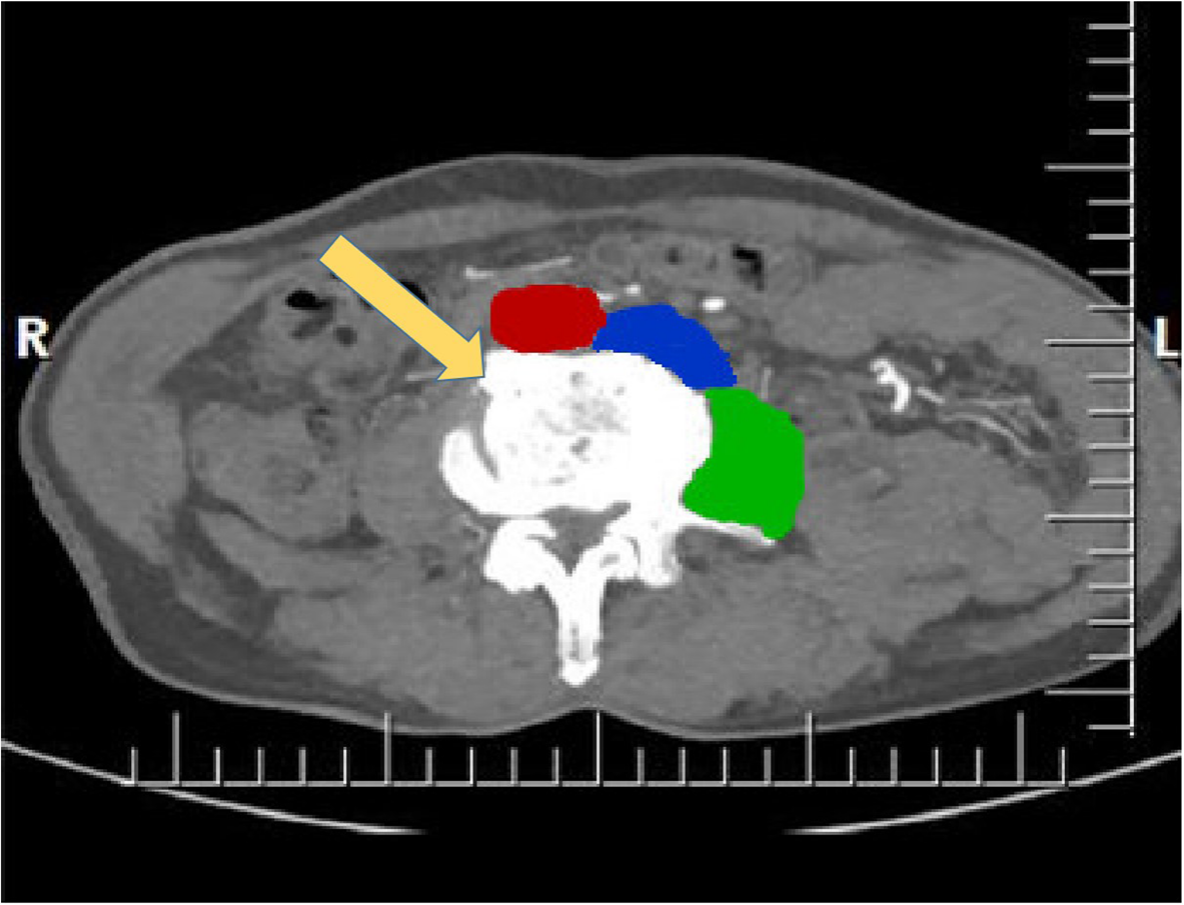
A transverse image of a contrast-enhanced abdominal computed tomography scan. The *red region* refers to the abdominal aorta; the *blue region* represents the inferior vena cava; and the *green region* represents the psoas major muscle. The *yellow arrow* represents the operation window
